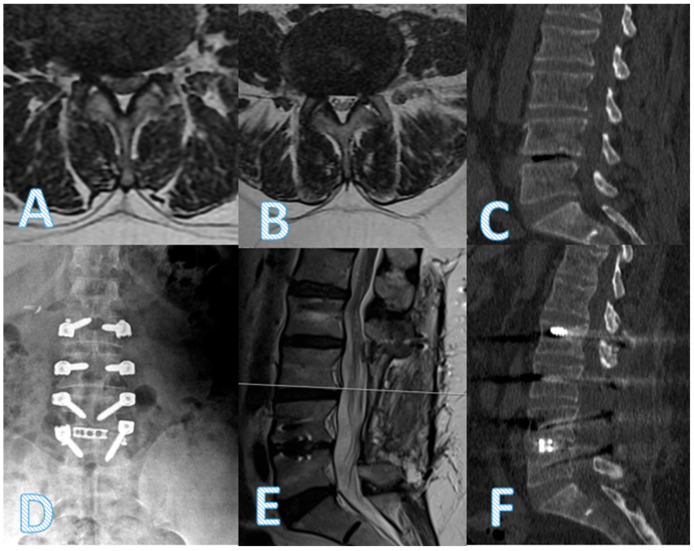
A 58-year-old female patient presented with low back pain (VAS 7) and right-dominant leg pain (VAS 8). Axial MR images at the L3–4 (A) and L4–5 (B) levels reveal bilateral foraminal stenosis, more severe at L4–5. Preoperative sagittal CT (C) shows disk space narrowing and endplate sclerosis at L4–5. Postoperative anteroposterior X-ray (D), sagittal T2-weighted MRI (E), and sagittal CT (F) demonstrate adequate decompression and stabilization following L4–5 transforaminal lumbar interbody fusion (TLIF) with a PEEK rod and interbody cage.
